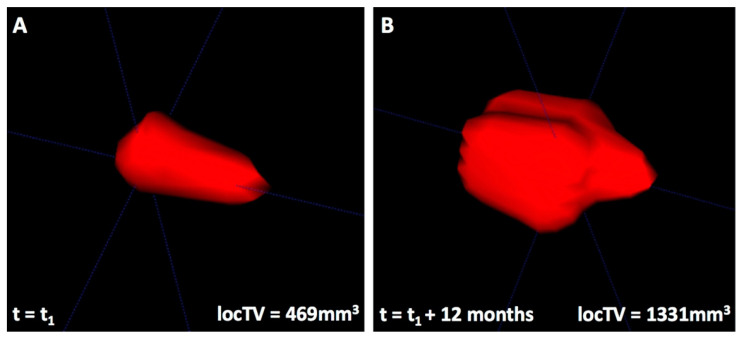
3D spatial tumor development of a focal lesion over time (t) in a female patient (55 years at t = t_1_). Segmented focal lesion images were acquired in a T2-weighted sequence and visualized by ITK-SNAP 3D-Viewer with relative scale and orientation. The presented focal lesion is located in the sacrum and shows a volume (V) of 469 mm^3^ in (A) and of 1331 mm^3^ 12 months later in (B), corresponding to a speed of growth of 2.36 mm^3^/day.
